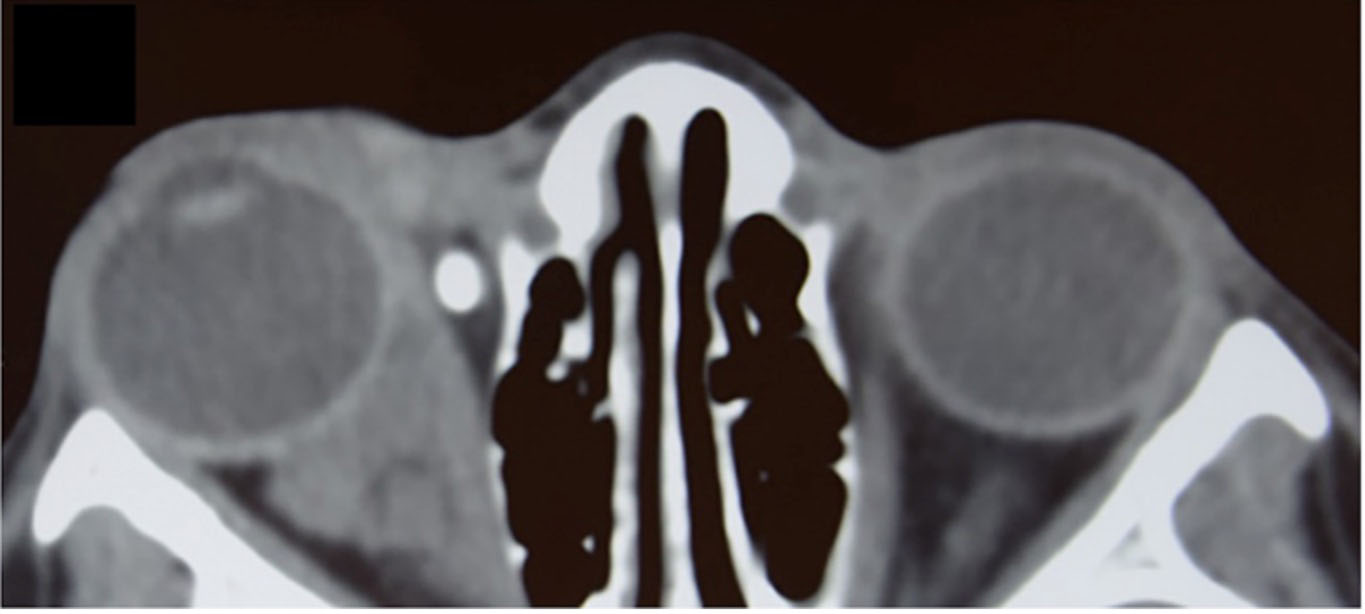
Axial non-contrast CT of the orbits in a 12-year-old girl demonstrating a lobulated, mostly iso-attenuating mass in the right orbit with both intraconal and extraconal components, including nasal preseptal extension. The lesion is causing mild globe displacement without bony erosion. A phlebolith is noted in the anteromedial orbit, consistent with a slow-flow vascular lesion and supporting a venous component within the overall lesion. Therefore, the lesion is classified as a lymphatico-venous malformation. Diagnosis was reached by further assessment and imaging.
